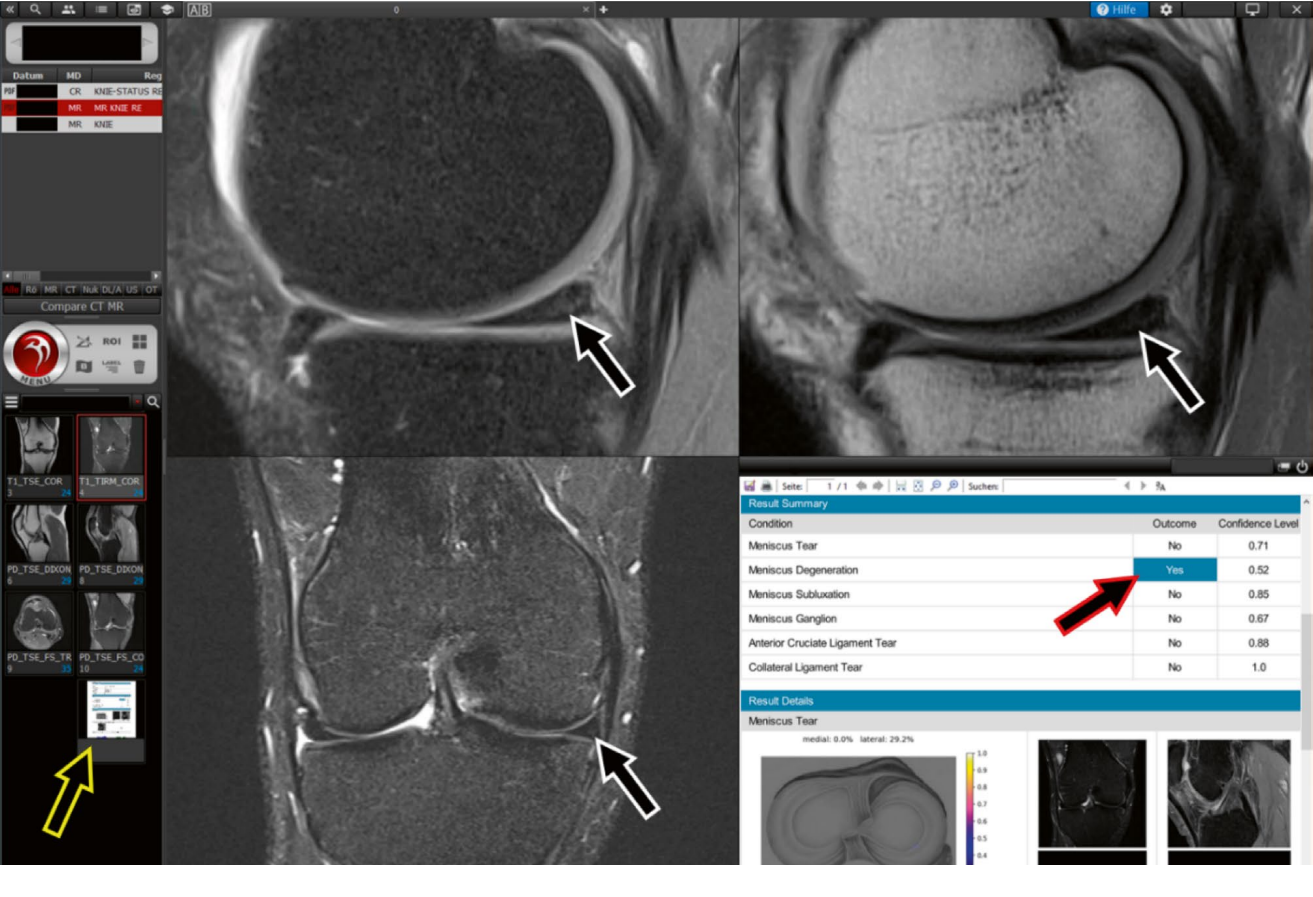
Artificial intelligence (AI)-augmented knee MRI interpretation using an investigational AI algorithm. The AI report appears as part of the MRI study in the left column (yellow-framed arrow) and can be displayed in a viewport (lower right viewport) or separate window (not shown). In this patient, the AI algorithm predicted internal degeneration of the medial meniscus with a probability of 52% (red-framed arrow), based on the intrasubstance signal hyperintensities (white-framed arrows), as well as absent meniscus tear, meniscus extrusion (subluxation), meniscus ganglions cyst, anterior cruciate ligament tear, and medial collateral ligament tear
